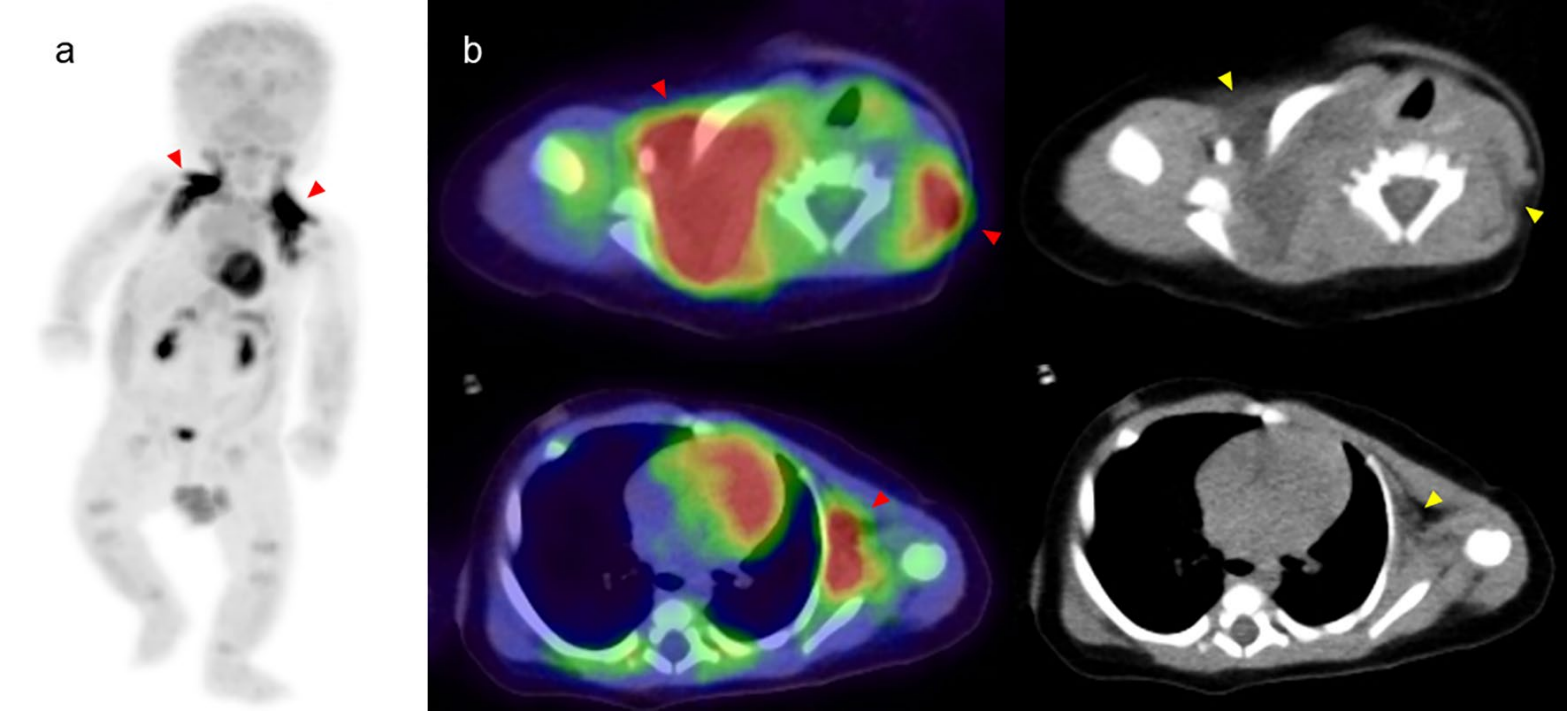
a Whole body FDG-PET image of infants with 51days after birth, b fused FDG-PET/CT, c CT portion of PET/CT. Significant FDG uptake is confirmed at bilateral neck, subclavian region and axillar region (red arrowhead) of those are corresponded to brown adipose tissue (yellow arrowhead)
